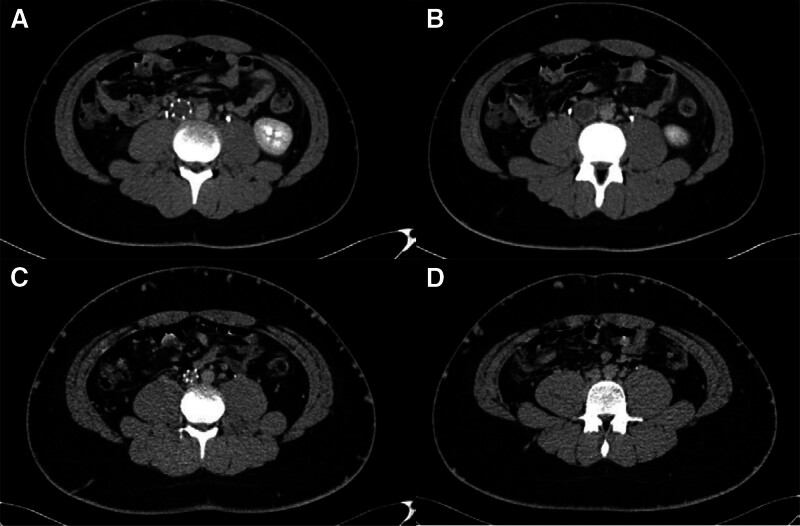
Comparison of computed tomographic venography (CTV) before and after medication. Figure (A) and (B) are before medication while (C) and (D) are after medication.
